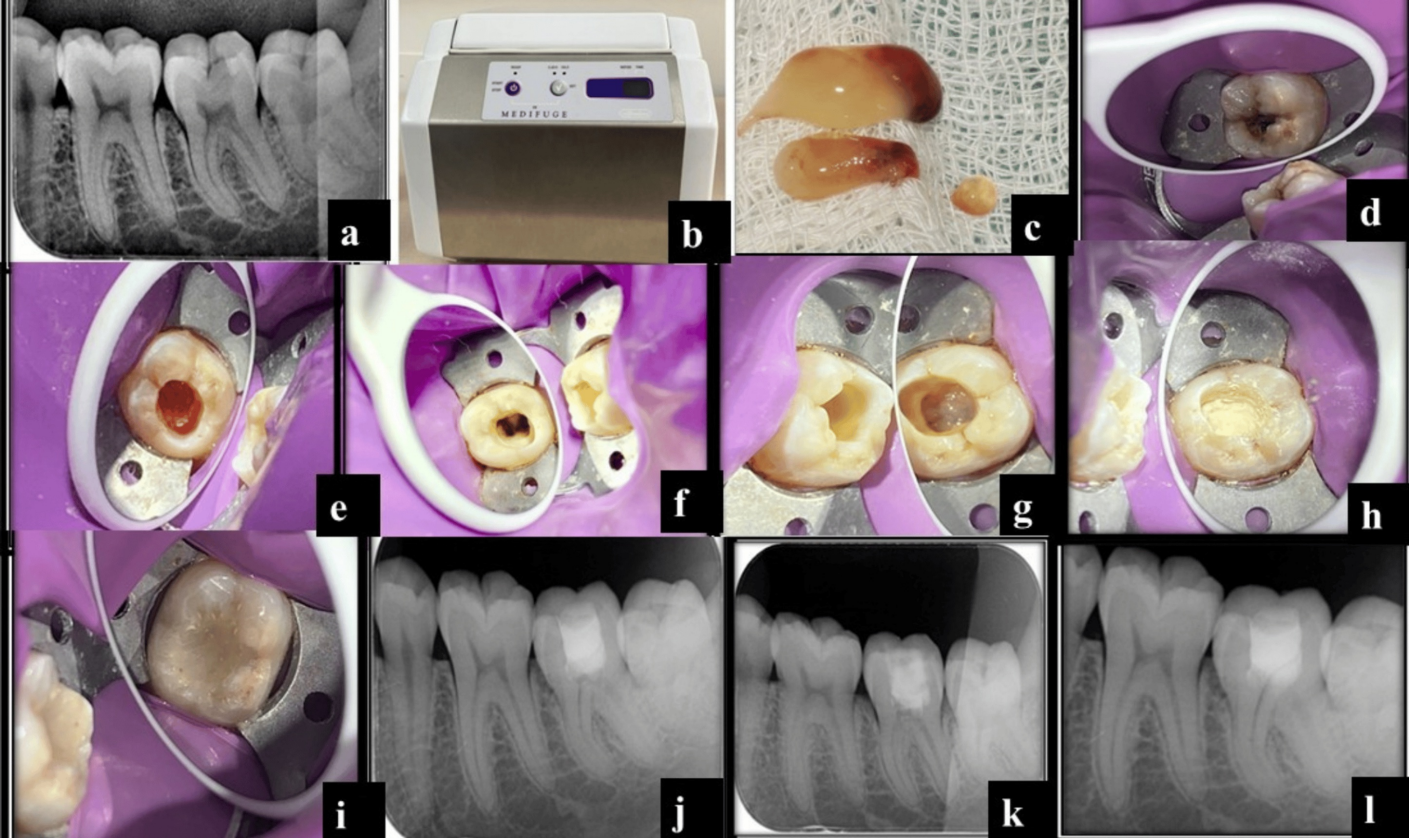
Preoperative radiograph (a); CGF machine (b); prepared CGF (c); clinical picture of the affected tooth (d); removal of coronal pulp (e); hemostasis achieved (f); placement of CGF membrane (g); placement of MTA (h); final restoration (i); postoperative radiograph (j); 6-month follow-up radiograph (k); 12-month follow-up radiograph (l) MTA: mineral trioxide aggregate
